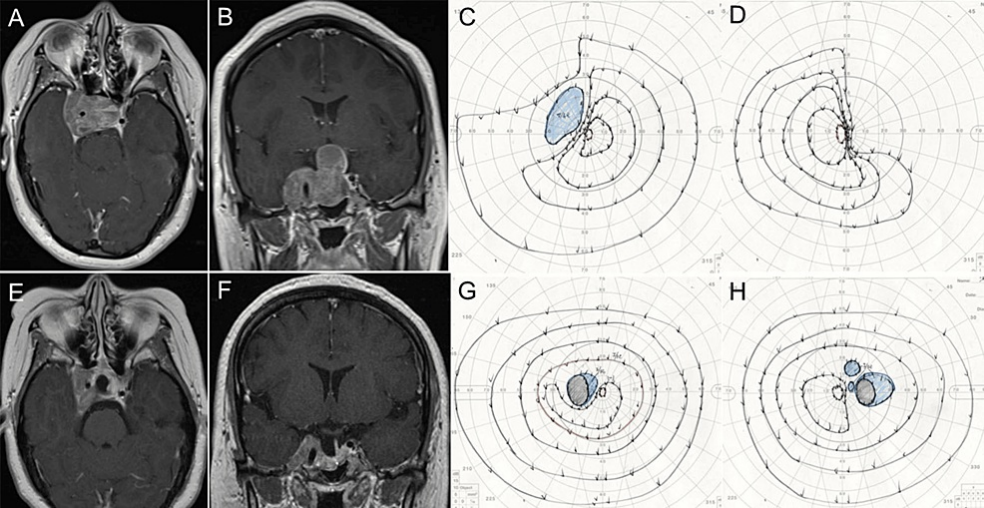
MRI images and visual field test obtained in a 35-year-old woman presenting with right oculomotor palsy and visual field loss. (A, B) Axial and coronal MRI shows a giant intra- and suprasellar tumor extending to the middle fossa (Knosp grade 4). (C, D) Preoperative visual field test shows defects in the upper temporal regions of both eyes. (E, F) Axial and coronal MRI postoperatively shows a residual tumor in the cavernous sinus. (G, H) Preoperative visual field test shows the improvement of visual field defects.
